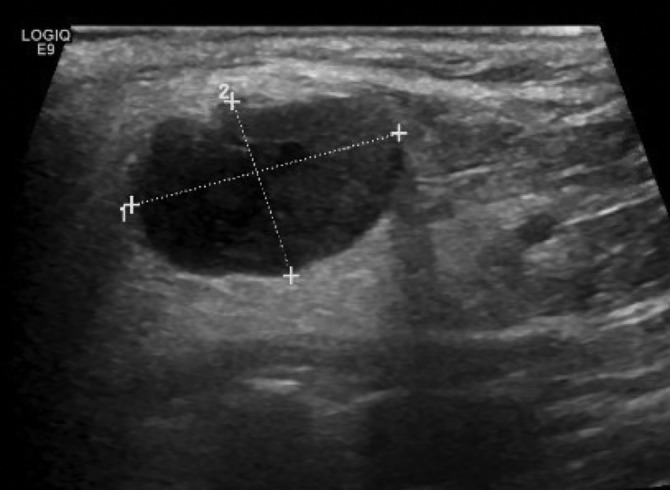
Ultrasound of parotid mass
